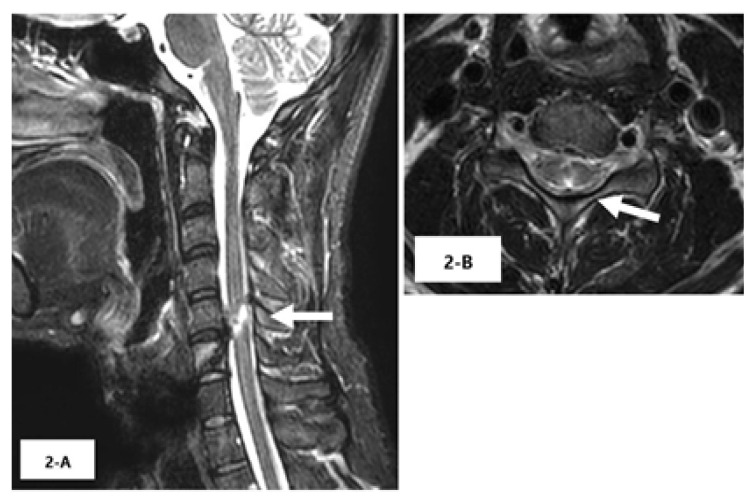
Postsurgical cervical magnetic resonance imaging showing high T2 signal intensity at the posteromedial region of the sixth cervical level, without any evidence of corticospinal tract damage (arrows).
